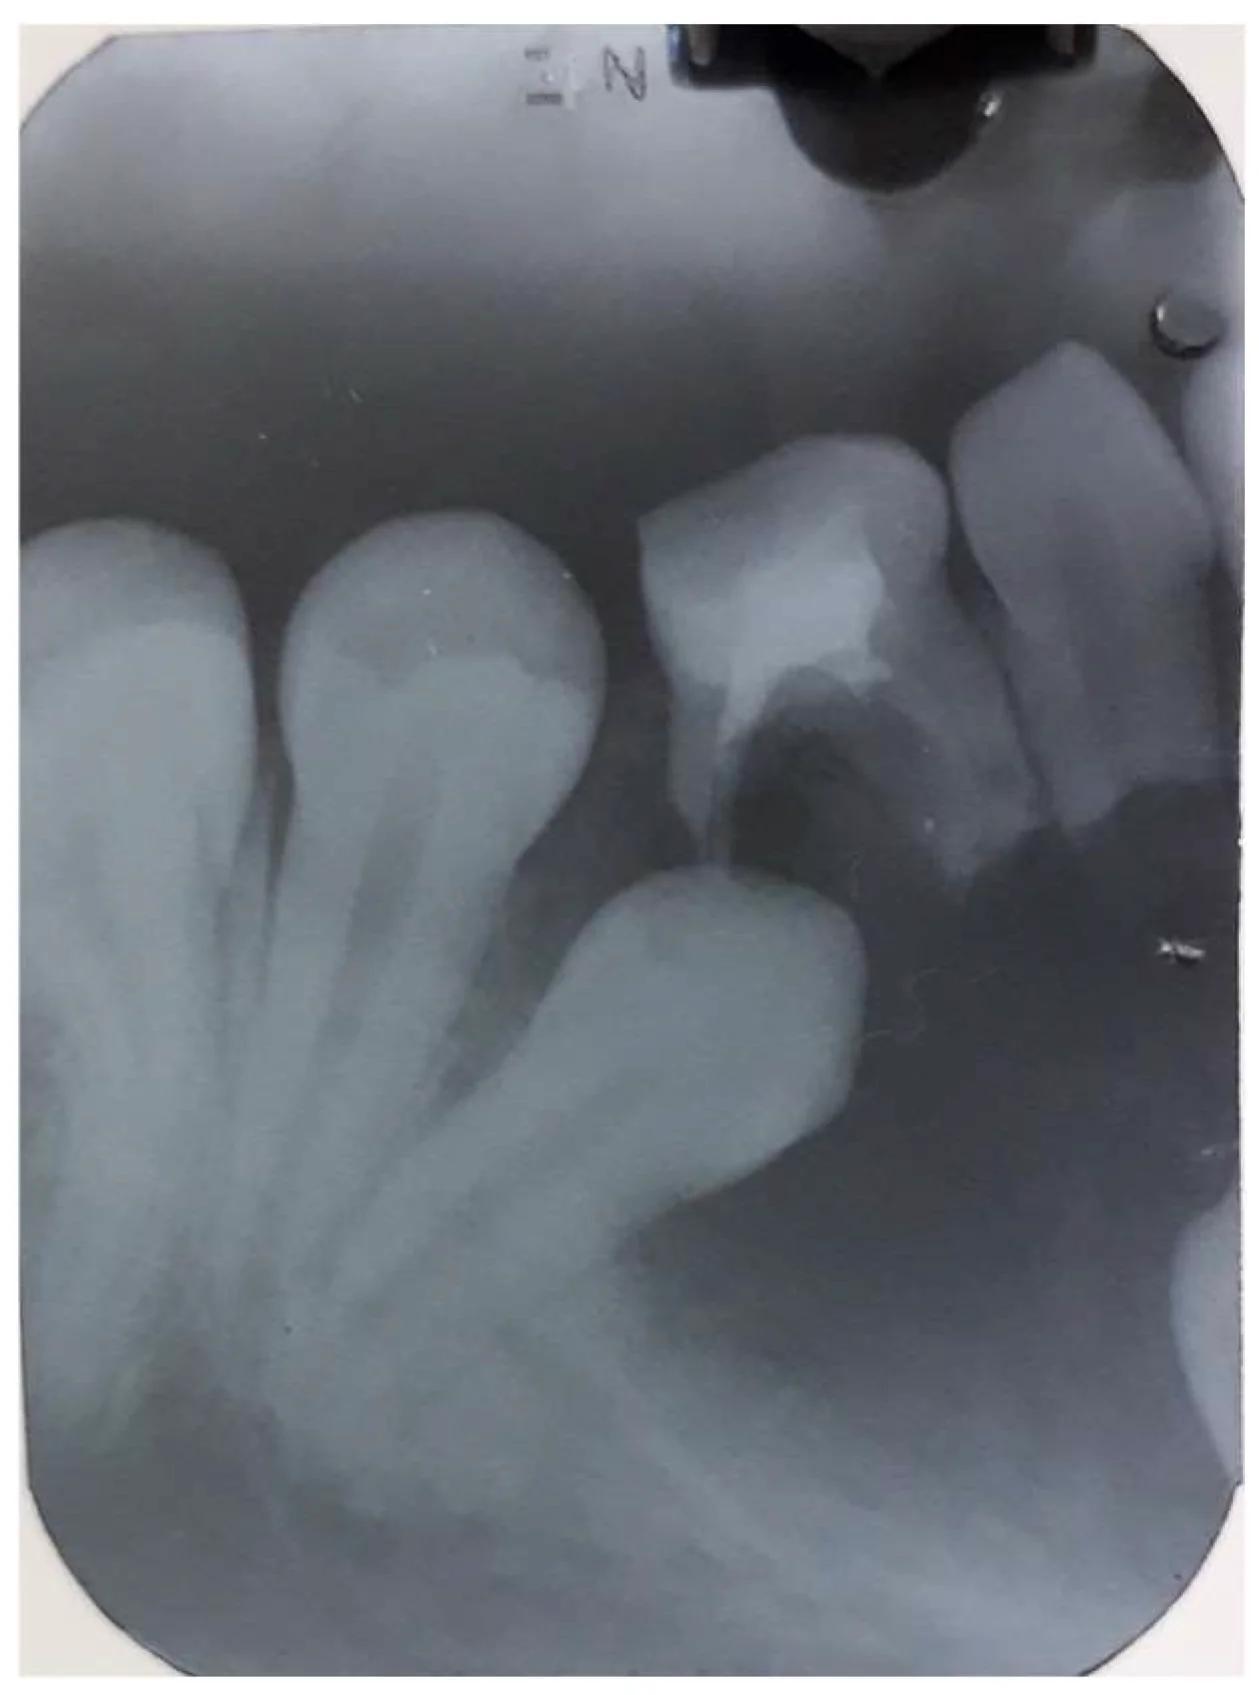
Pretreatment periapical radiograph.
